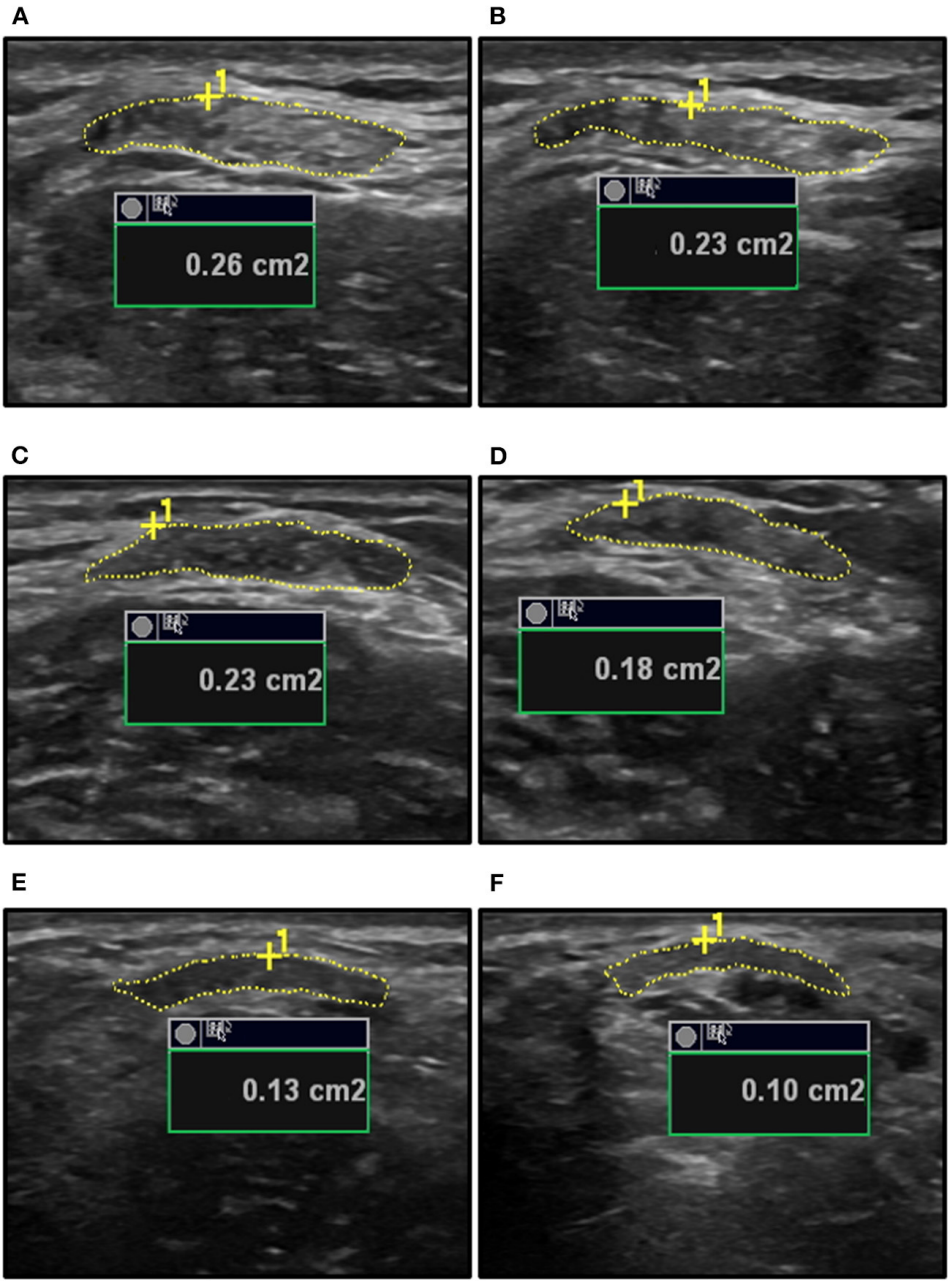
The ultrasound measurements of biceps brachii (BB) tendon cross-sectional area (CSA) for a young male at rest (A) and during a 20% MVC contraction (B), an old male at rest (C) and during a 20% MVC contraction (D), and a young female at rest (E) and during a 25% MVC contraction (F). The inherent measurement platform of the ultrasound provides the area in square centimeters (cm^2^) and this was converted to square millimeter (mm^2^) prior to the calculations of tendon stress using the resting and the instantaneous tendon CSA measures. MVC, maximal voluntary contraction.
